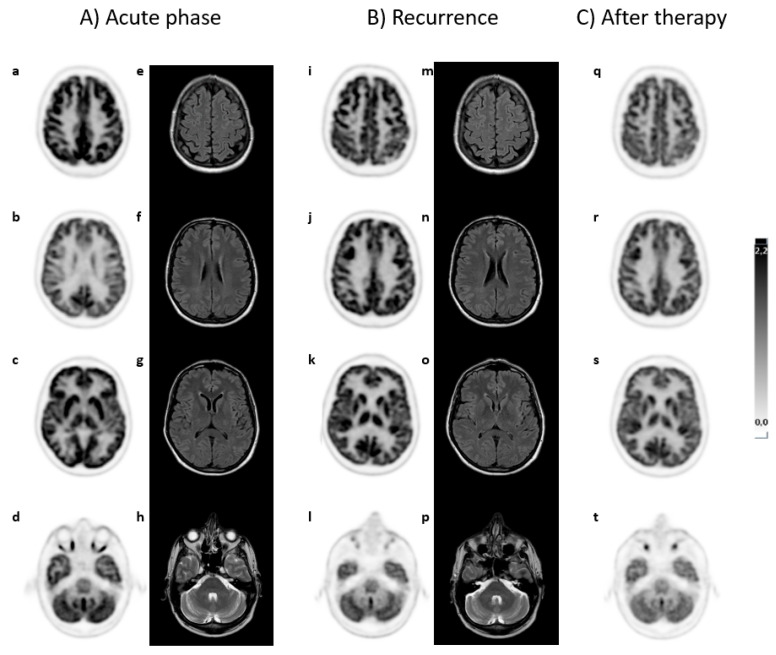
(A) A 52-year-old male patient (case G) in the acute phase of autoimmune encephalitis (AE) presented with proteinorrhachia, three IgG bands, and later anti-GAD65 antibodies in cerebrospinal fluid (CSF). A brain ^18^F-FDG PET/CT scan, in transaxial view, indicated increased FDG uptake in the cerebellar vermis (d), while other areas (a–c) exhibited normal uptake. MRI T2/FLAIR (e–h) findings were nonspecific. (B) Six months after the initial acute episode, during which the patient was treated with methylprednisolone and immunoglobulin, a recurrence occurred. A repeat brain ^18^F-FDG PET/CT showed new areas of hypermetabolism in the fronto-parietal (i,j) and parieto-temporal (k,l) cortical regions bilaterally, as well as persistent hypermetabolism in the cerebellar vermis (m). The MRI results remained nonspecific (n–p). (C) After a second therapy cycle, a new brain ^18^F-FDG PET/CT scan indicated no significant change in the hypermetabolic cortical areas (q–s), while there was a modest reduction in FDG uptake in the cerebellar vermis (t). No MRI was performed at this time. The patient is still alive and remains under observation.
